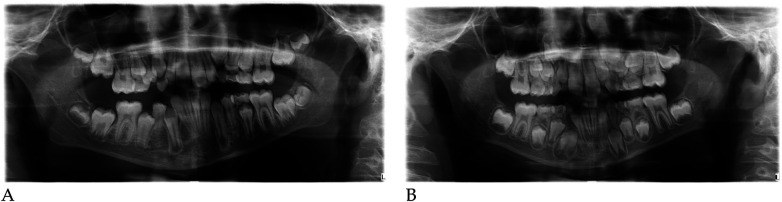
Pre- (A) and post-treatment (B) panoramic radiographs of case 7.
